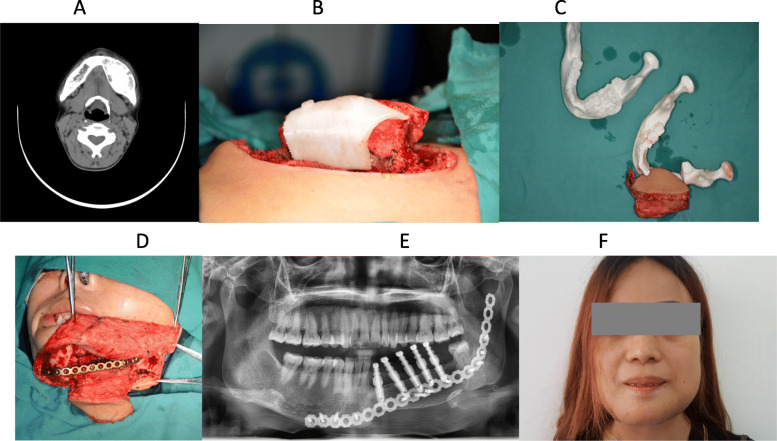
a The CT in the maxillofacial region shown that the bone enlargement, destruction, and irregular mass of the mandible with a larger scope of approximately 5.5*3.1 cm; b Preparation of vascularized free iliac musculocutaneous flap; c Comparison of the prepared iliac bone flap with the 3D model; d Use of prefabricated titanium plates for fixing the disconnected mandible and the intercepted iliac bone; and e The second stage of dental implants; f The facial appearance and occlusion function after follow-up
